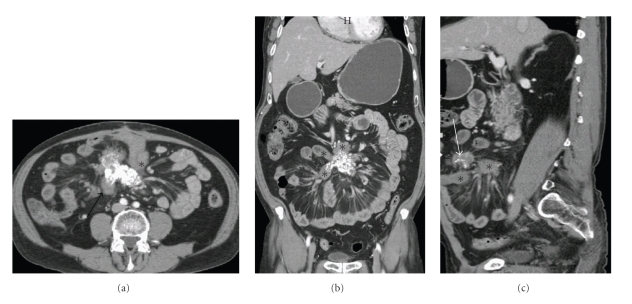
Sclerosing mesenteritis*. (a) Contrast enhanced CT Axial section through the mid abdomen shows a heterogeneous soft tissue density (black arrow) with calcifications in the root of mesentery and mesenteric fat stranding with fibrotic kinking and traction over small bowel loops as shown by an asterisk. (b) Contrast enhanced CT coronal section redemonstrating fibrosing reaction in the mesentery with pulling of adjacent bowel loops. (c) Contrast enhanced CT sagittal section clearly showing speculation in the mesenteric mass (straight white arrow) with mesenteric fat infiltration.
